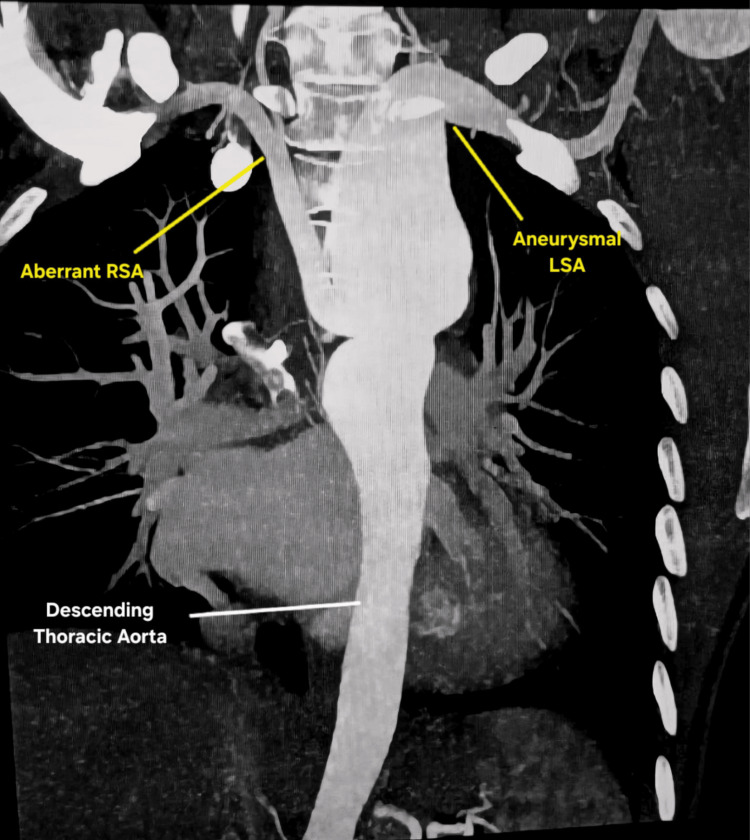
Coronal CT angiography showing aneurysmal dilatation (~34 mm) of the left subclavian artery (LSA) arising from the aortic arch. There is also an anomalous right subclavian artery (RSA) (arteria lusoria).
